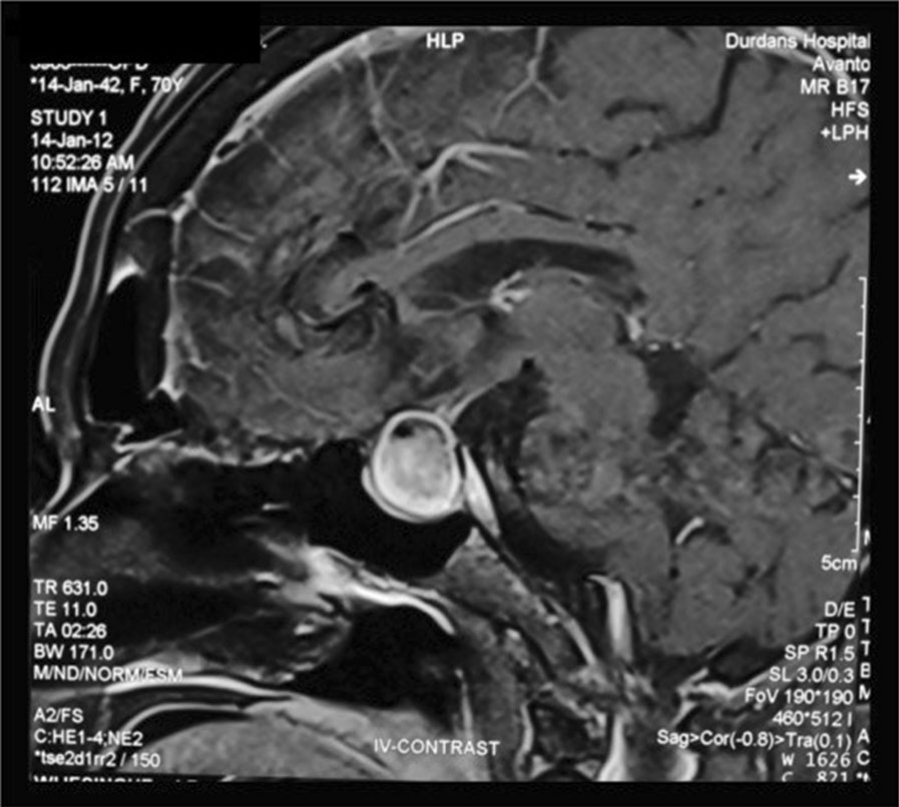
Suprasellar region mass seen in magnetic resonance imaging
